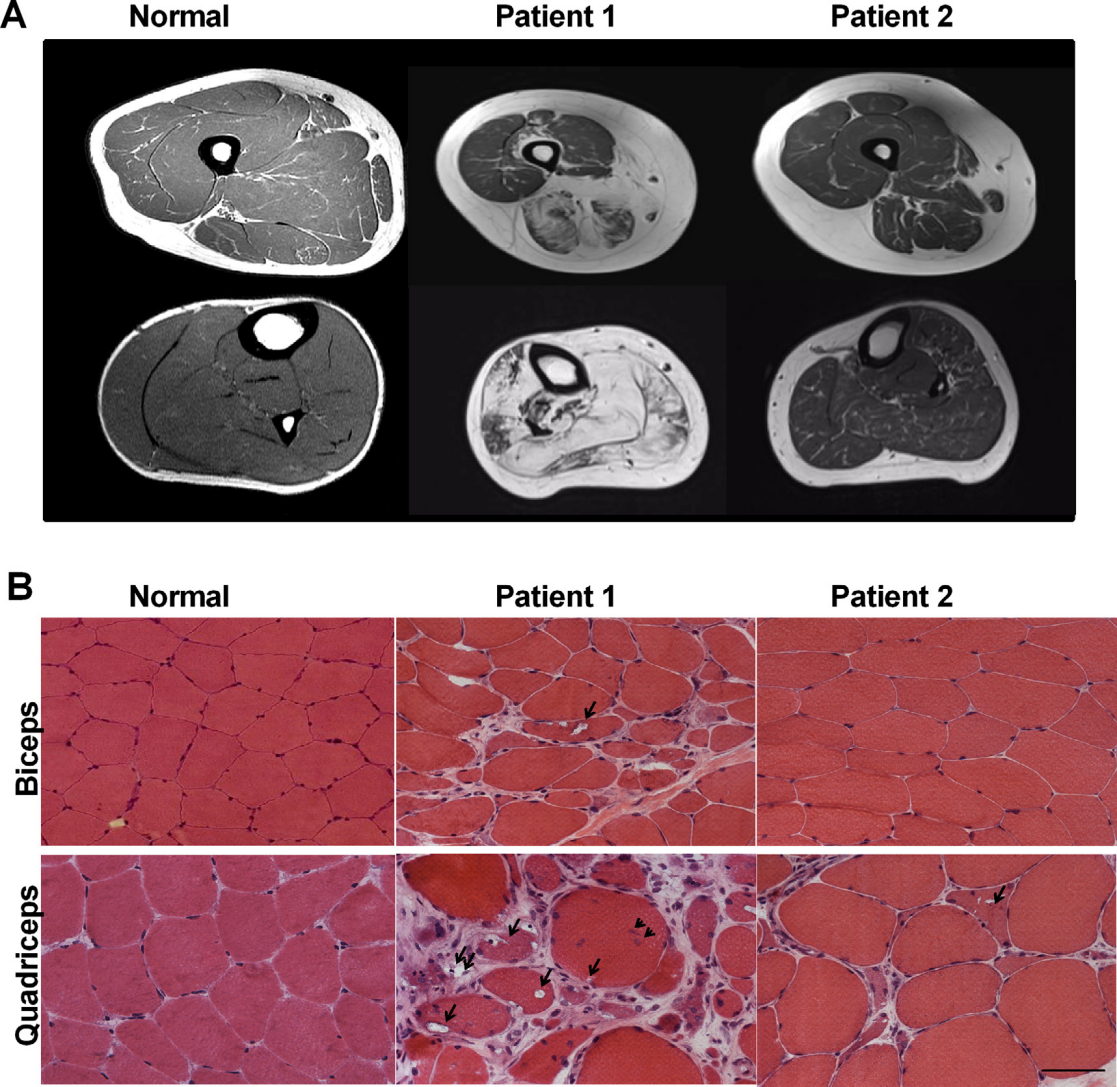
Muscle imaging and pathology. (A) T_1_‐weighted MRI of the thigh (*upper panel*) and lower leg (*lower panel*). Atrophy of hamstrings and lower leg muscles (Patient 1) and the proximal anterior tibialis (Patient 2) gave rise to fatty infiltration, apparent as white on the MRI. (B) Muscle biopsies of biceps brachii and lower extremity muscles (gastrocnemius medialis in Patient 1, anterior tibialis in Patient 2, quadriceps femoris in Normal) show characteristic findings of GNE myopathy, including rimmed vacuoles (*arrows*), fatty and fibrous tissue replacement (*double arrows*), marked variation in fiber size, and central nucleation (*arrowhead*). Note that the biceps muscle of Patient 2 appears normal, except for a mild variation in fiber size. Scale bar = 50 microns.
